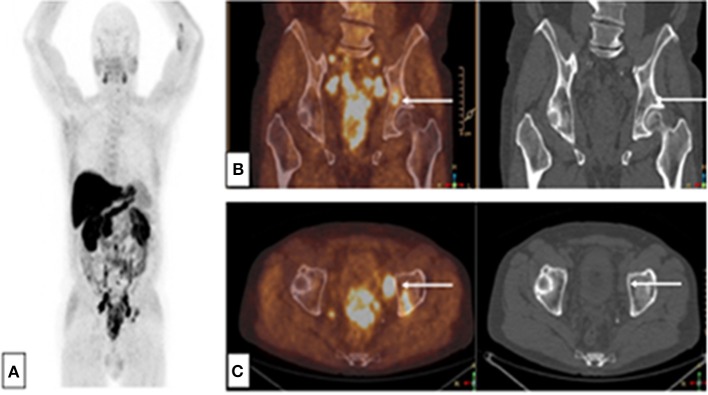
Choline-PET-CT: Single bone lesion of the left acetabulum, without CT abnormality. Initial assessment of a patient with immediately metastatic prostate adenocarcinoma with bone and node lesions. Gleason 8 (4 + 4), cT3, PSA = 36 ng/mL, (A) MIP reconstruction, (B) PET-CT and CT frontal slices, (C) PET-CT and CT axial slices.
